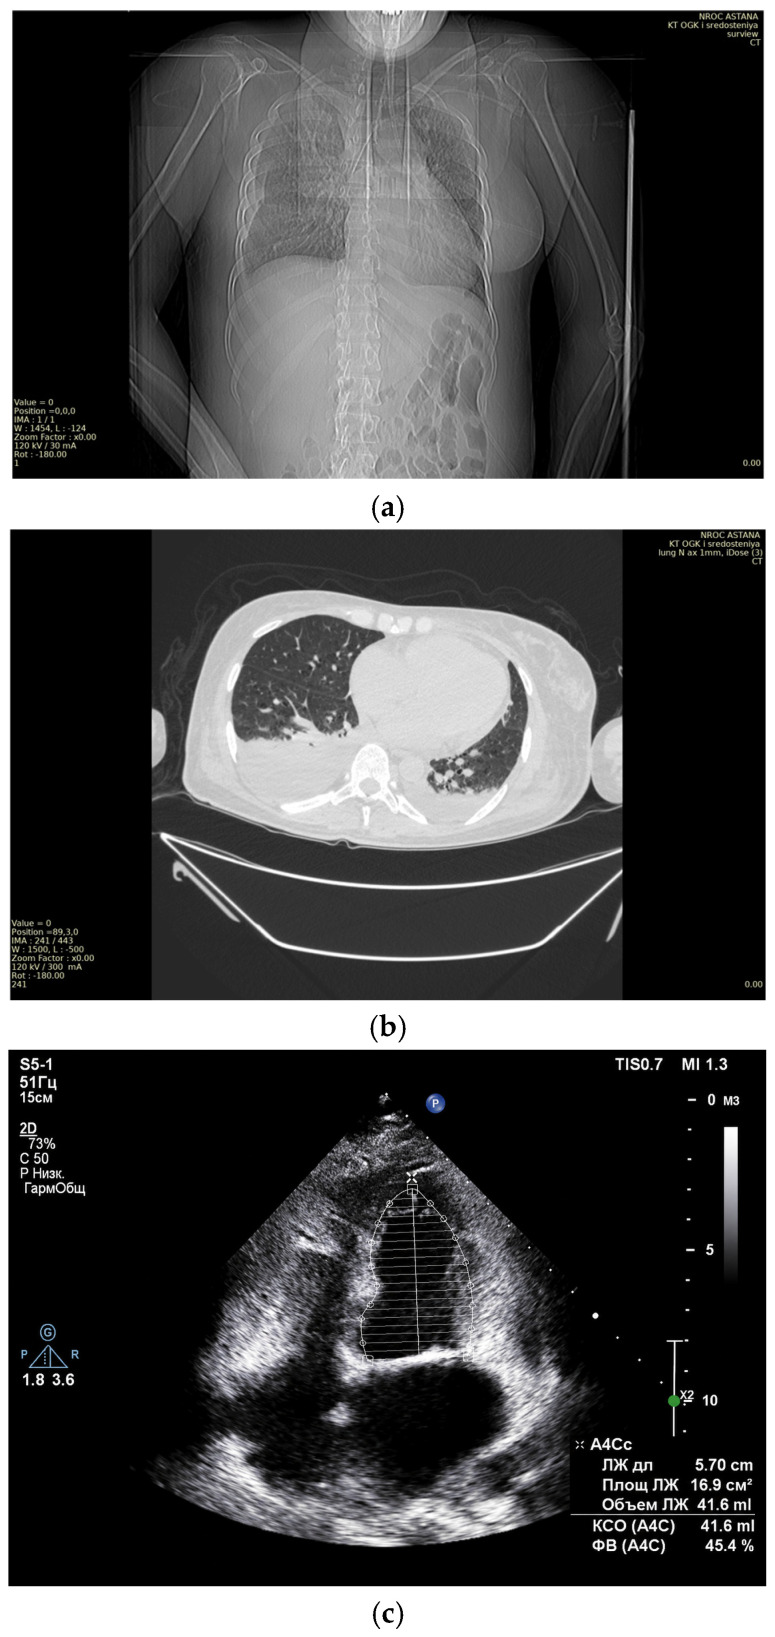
Imaging findings during early post-transplant complications. (a) Chest computed tomography demonstrating bilateral pleural effusion and signs of fluid overload. (b) Chest X-ray confirming pulmonary congestion. (c) Transthoracic echocardiography (apical four-chamber view) showing reduced left ventricular systolic function, with an ejection fraction of 45.4% and a left ventricular volume of 41.6 mL, consistent with cardiac involvement in multi-organ dysfunction.
